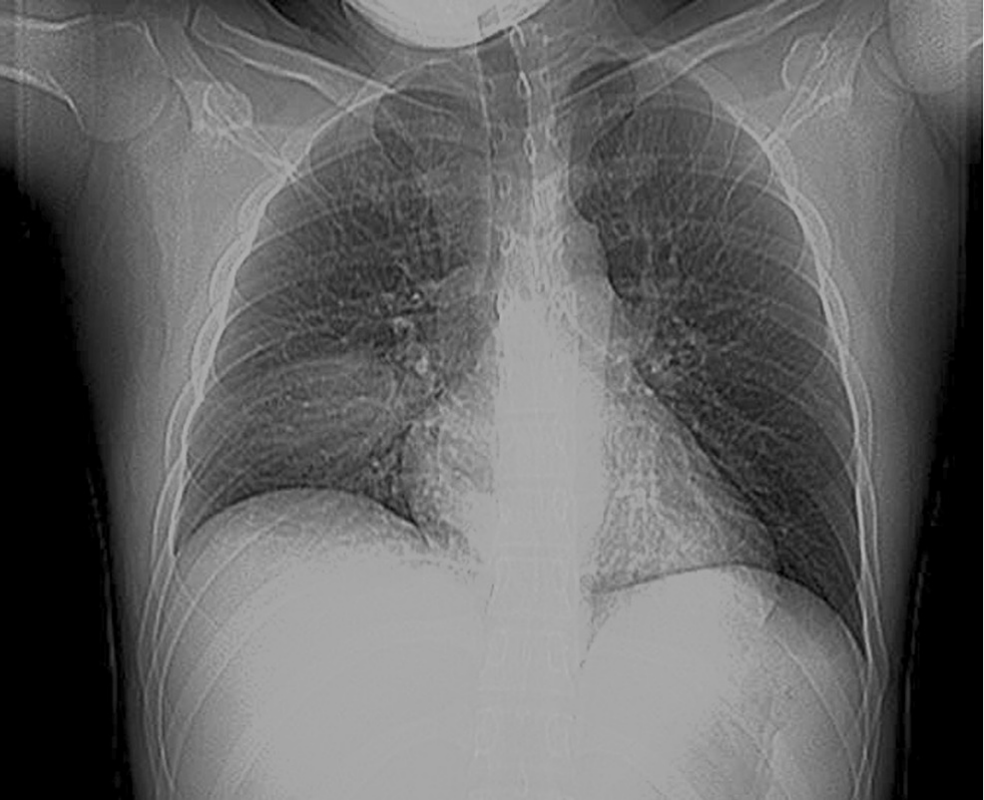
Portable X-ray of the chest. Portable chest X-Ray with an anteroposterior view demonstrating a consolidation in the right midlung, likely in the inferior right upper lobe. In this view, the left lung appears clear, likely due to the positioning of the patient.
